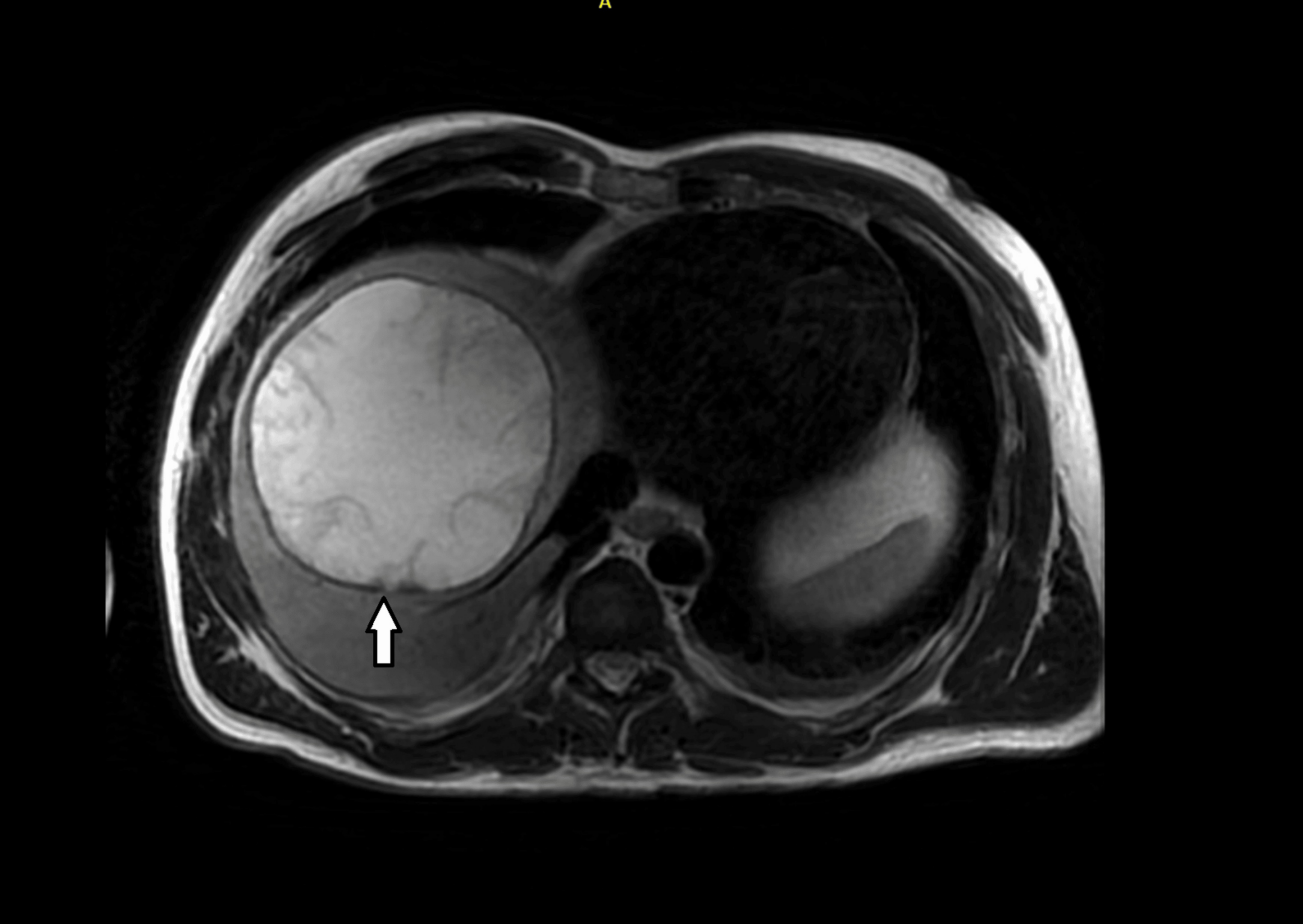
A magnetic resonance imaging scan with contrast of the abdomen A large solitary cystic liver lesion in the right lobe of the liver measuring 13 x 13 cm with thick and smooth wall enhancement; there are also some linear low-signal areas likely indicating septa, along with debris within the cyst (white arrow)
